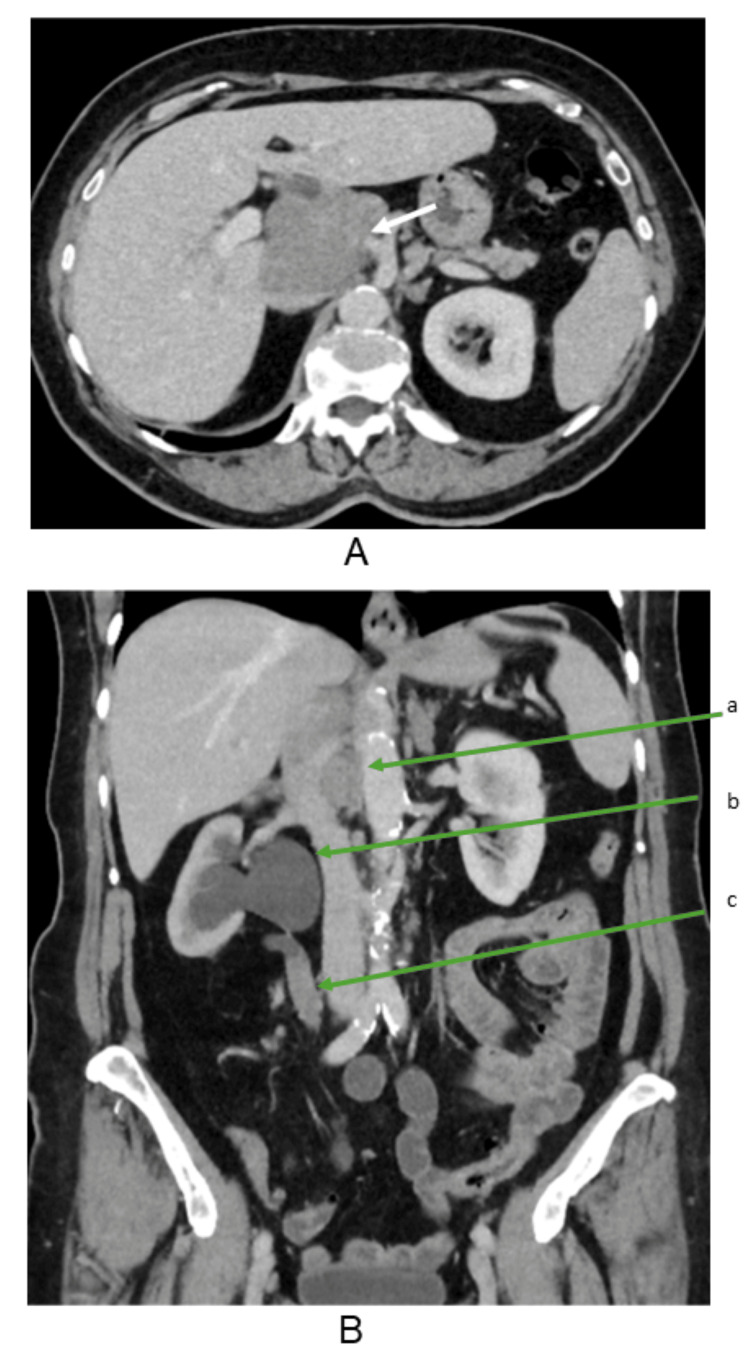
CT chest, abdomen, and pelvis (A) Axial CT image showing enlarged periportal lymph nodes (arrow). (B) Coronal CT image demonstrating (a) periportal lymph nodes, (b) right hydronephrosis, and (c) right ureteric soft tissue thickening CT: computed tomography
